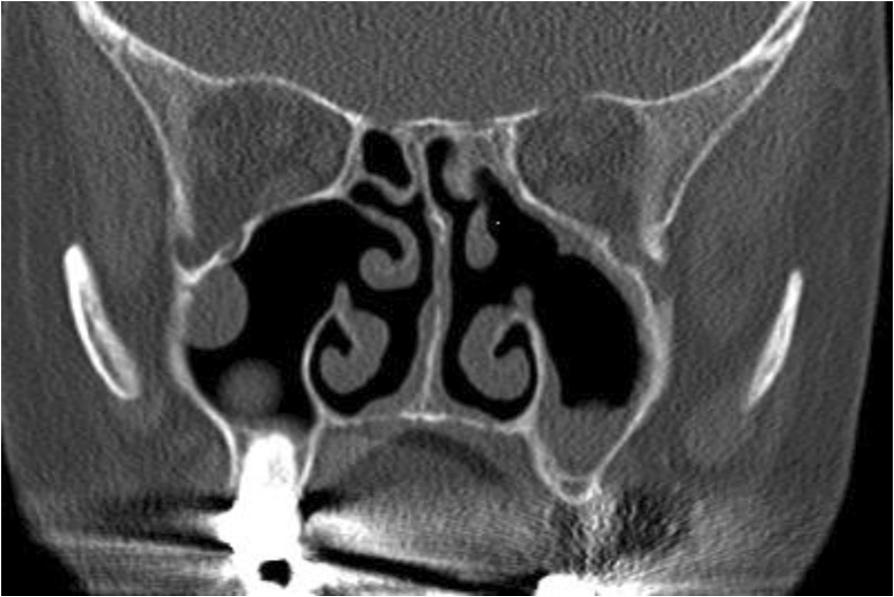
CT scan (coronal) of biopsy-proven sinonasal sarcoidosis affecting both maxillary sinuses.
